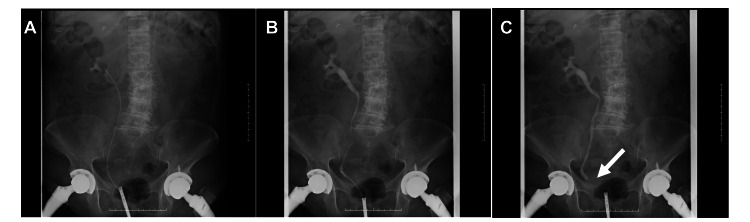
X-rays showing retrograde pyelogram (A) After removing the stones in the right urinary tract, the urinary catheter was advanced to the right renal pelvis. (B) The X-ray demonstrates the renal pelvis and right urinary tract. (C) Retrograde pyelogram after removal of the right ureteral stones showing temporary obstruction of the ureter (arrow).
